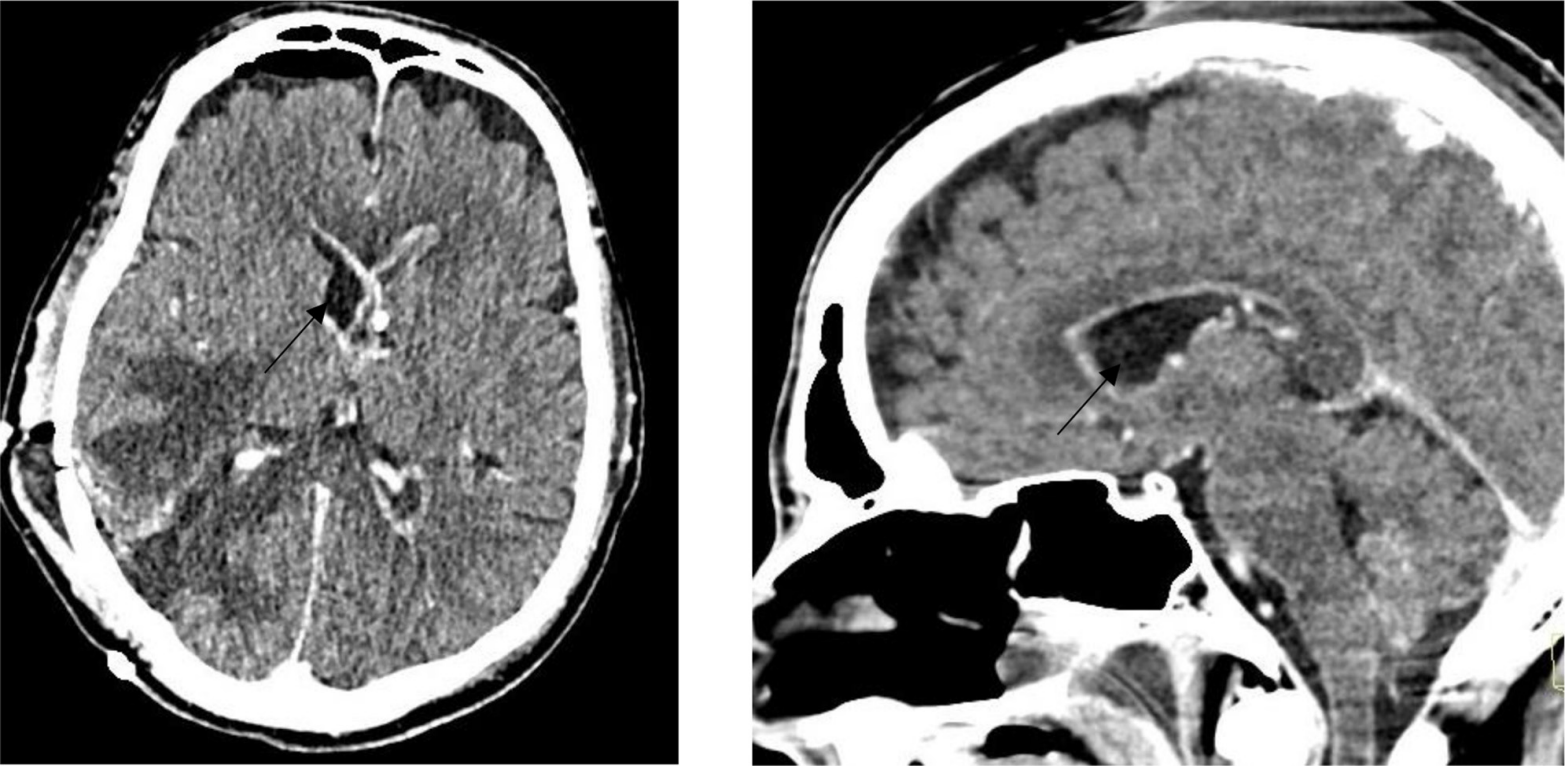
Post-operative brain contrast-enhanced computed tomography demonstrating the ventriculitis.
